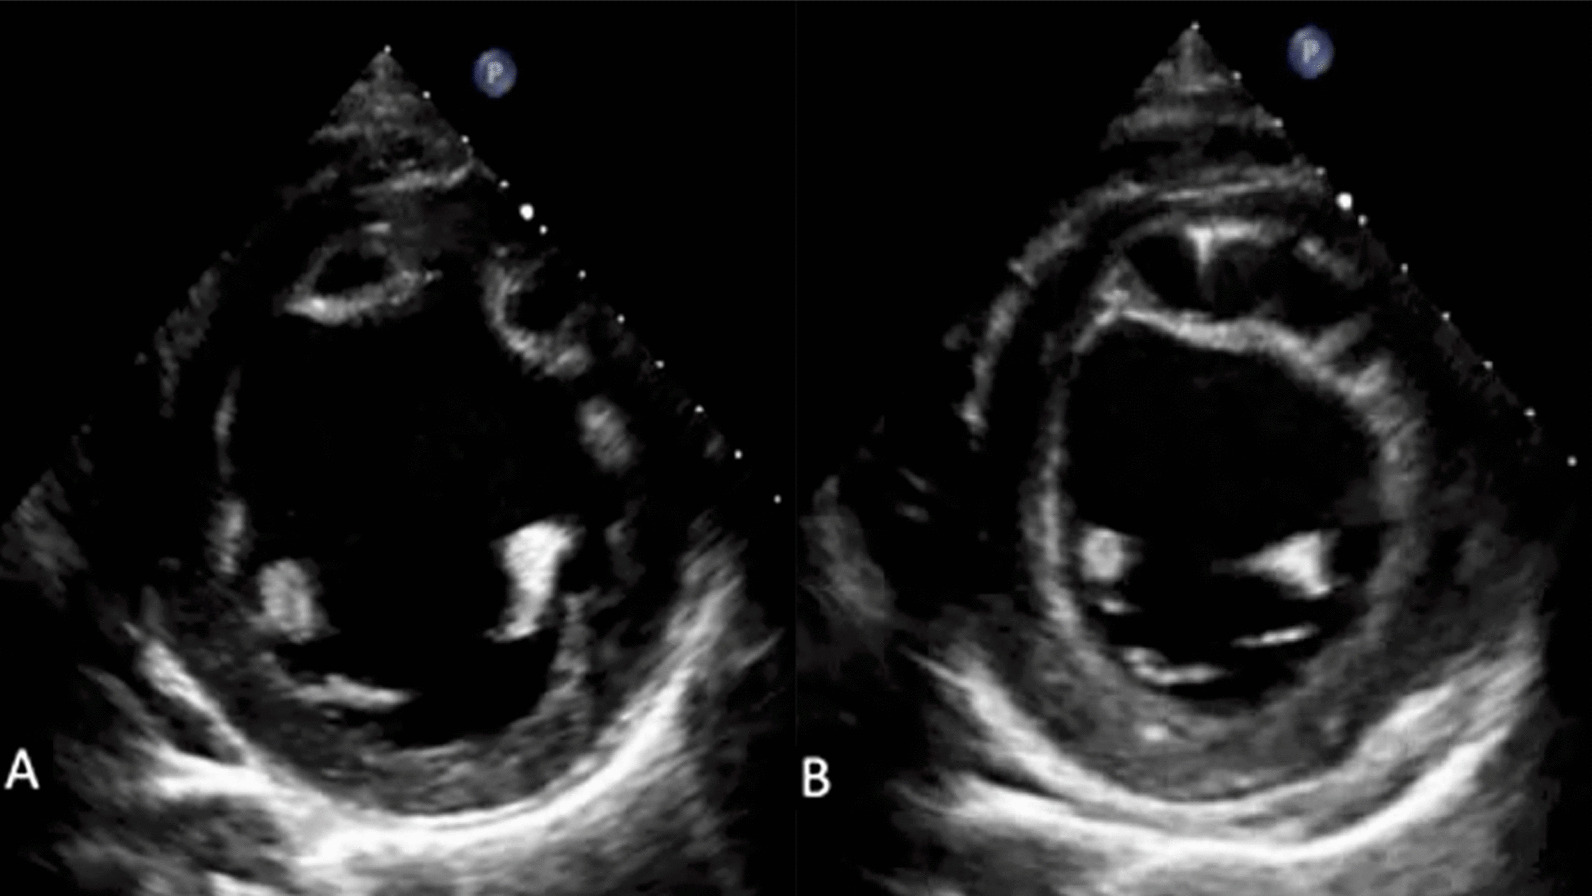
Transthoracic echocardiography. Echocardiography showed a cyst-like mass at the left anterior descending artery territory wall. The mass changed in size during diastole (a) and systole (b)
